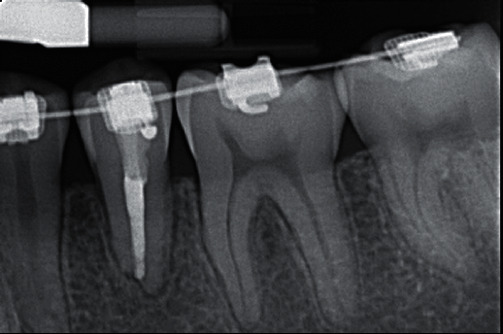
Periapical radiograph of tooth #20 at 8-month post-operative follow-up.
